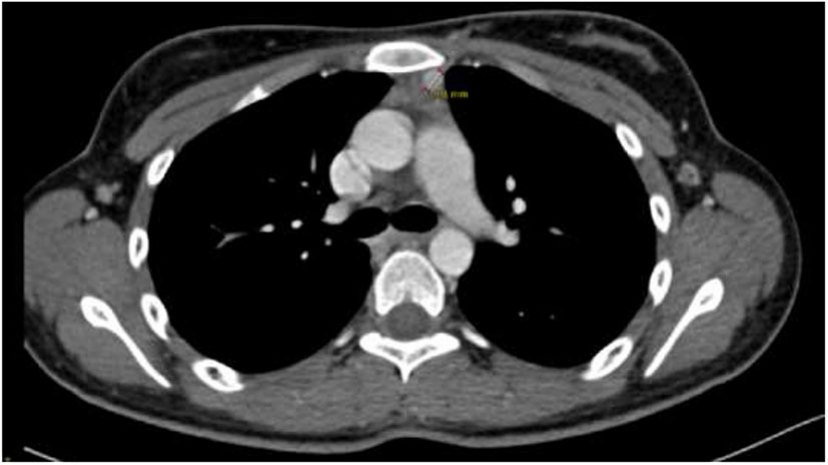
CT scan of the chest showing solid nodularity approximately 9 mm in diameter in the anterior mediastinum.
